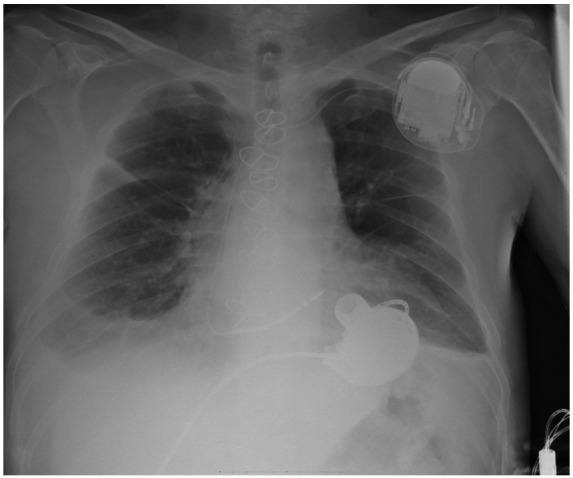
Chest radiograph on Day 0 did not show pulmonary opacity. Residual pleural effusion was due to the patient’s last pleural drainage.
